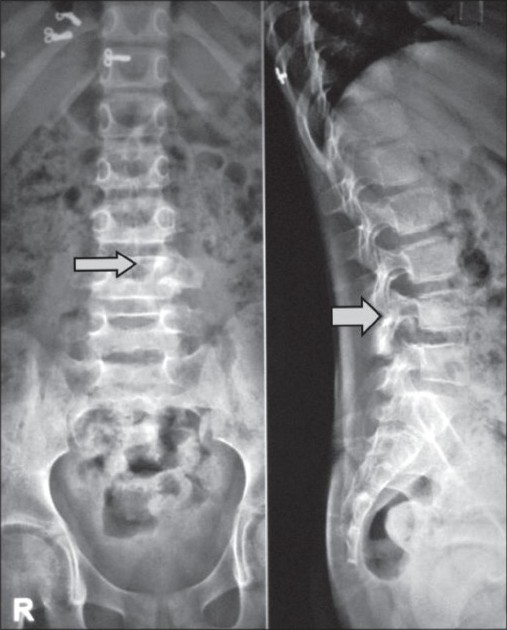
Plain X-ray showing expansion of the left fourth lumbar pedicle: transverse process and superior facet
